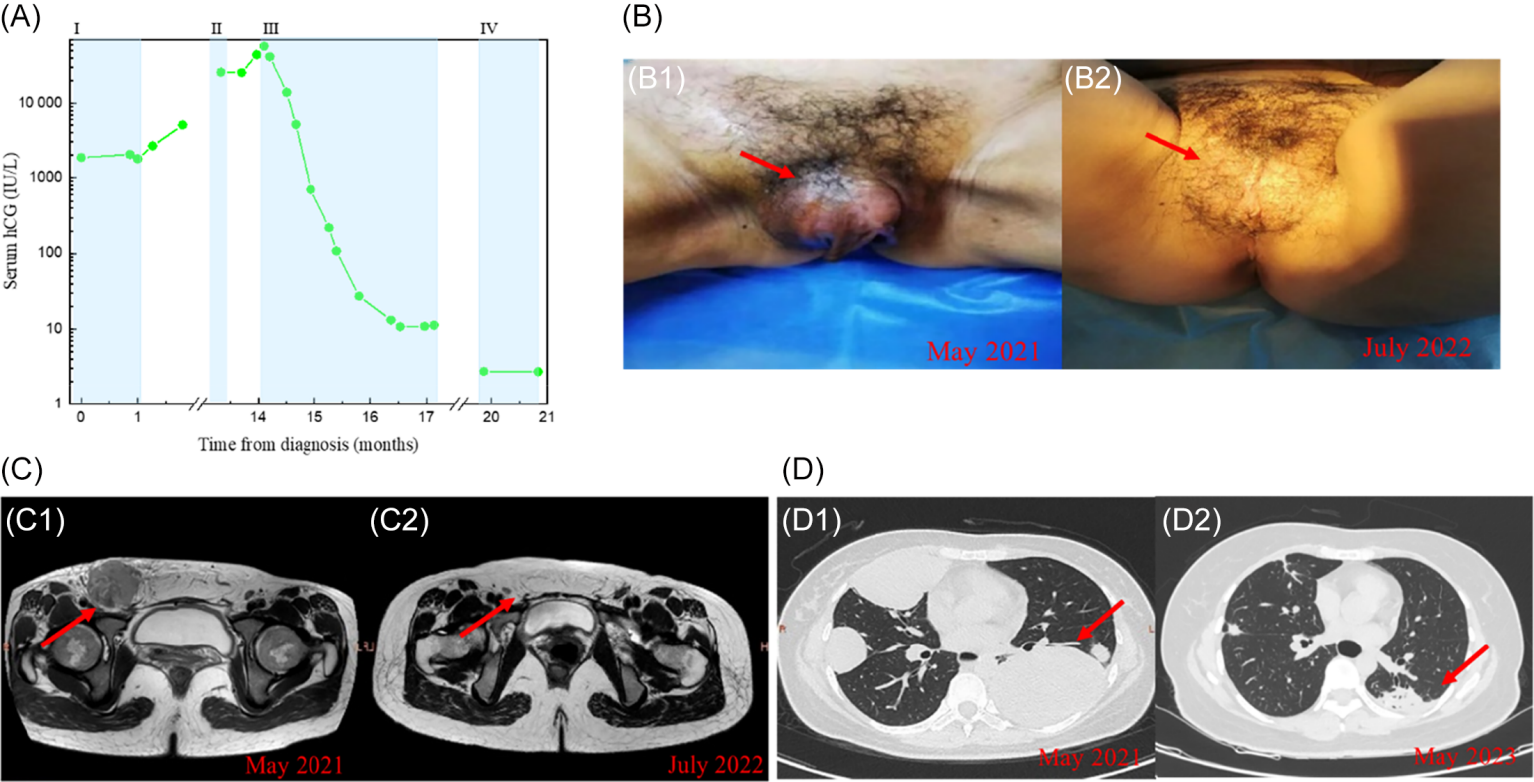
Clinical characteristics of patient 3. (A) Serum hCG is plotted against time from treatment initiation. Shaded bands represent duration of chemotherapy and immunotherapy with I, methotrexate; II, EMA‐CO; III, EP‐EMA + sintilimab; IV, TP/TE + sintilimab. (B) Gross appearance of the metastasis of vulva (arrow). B1: May 2021; B2: July 2022. (C) Pelvis enhanced scans show the reduction of metastases in vulva and right groin (arrow). C1: May 2021; C2: July 2022. (D) CT chest imaging shows resolution of a lung metastasis (arrow). D1: May 2021; D2: May 2023.
